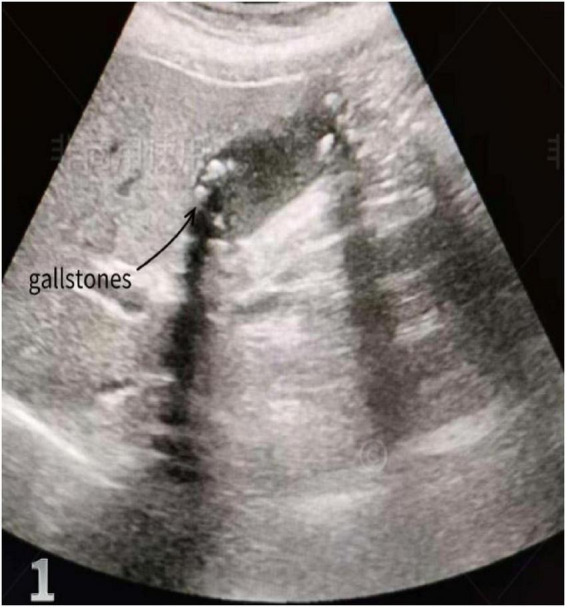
Ultrasound image showed gallstones.
